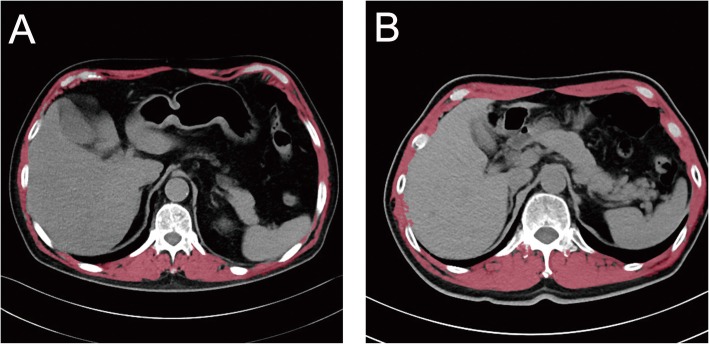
Thoracic SMI with CT of low skeletal muscle mass and normal skeletal muscle mass. Axial computed tomography images of the twelfth thoracic region, with skeletal muscle highlighted in red (40 to 80 Hounsfield units). (a) Example of an elderly male patient with a low T12 SMI (< 42.6 cm^2^/m^2^). (b) Example of an elderly male patient with a high T12 SMI (> 42.6 cm^2^/m^2^). SMI: skeletal muscle index, CT: computed tomography
